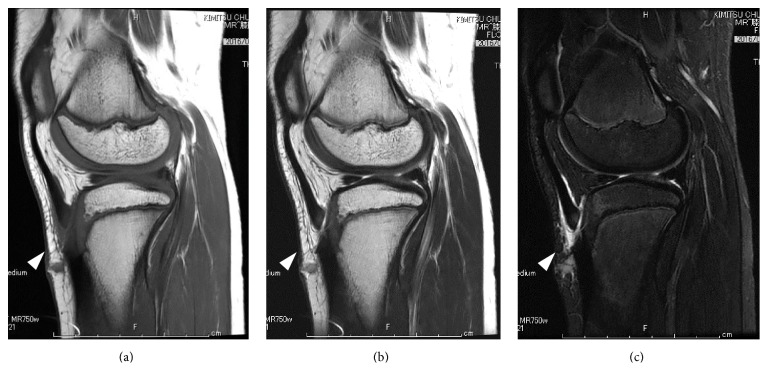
Preoperative lateral MRI of the left knee on (a) a T1-weighted image, (b) a T2-weighted image, and (c) a short T1 inversion recovery (STIR) image. The white arrowhead indicates a rupture of the patellar tendon.
